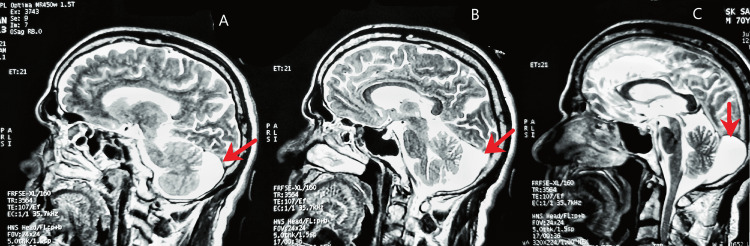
Head MRI (T2-weighted) in the sagittal plane
